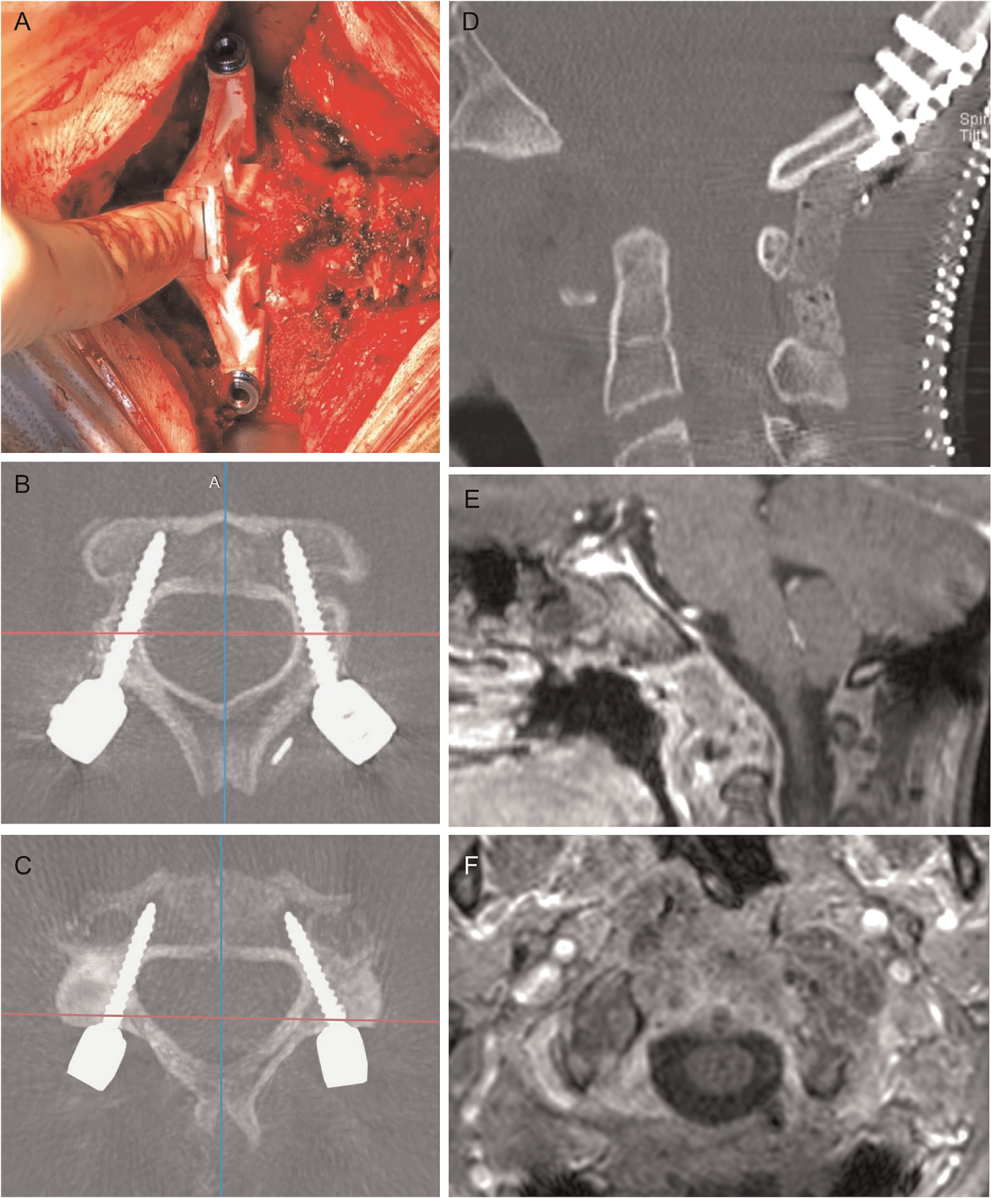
Intraoperative view of the printed guide fitting on C3 lamina (A) and postoperative results. Transversal CT scan of screws' trajectories in C2 and C3, respectively (B, C). The result of anterior decompression was shown in (D) and an occipital plate and bicortical screws connected with precontoured rods completed the occipitocervical constructs. One month brain and spine MRI (E, F) confirms the decompression of the cervicomedullary junction and the optimal extent of resection.
